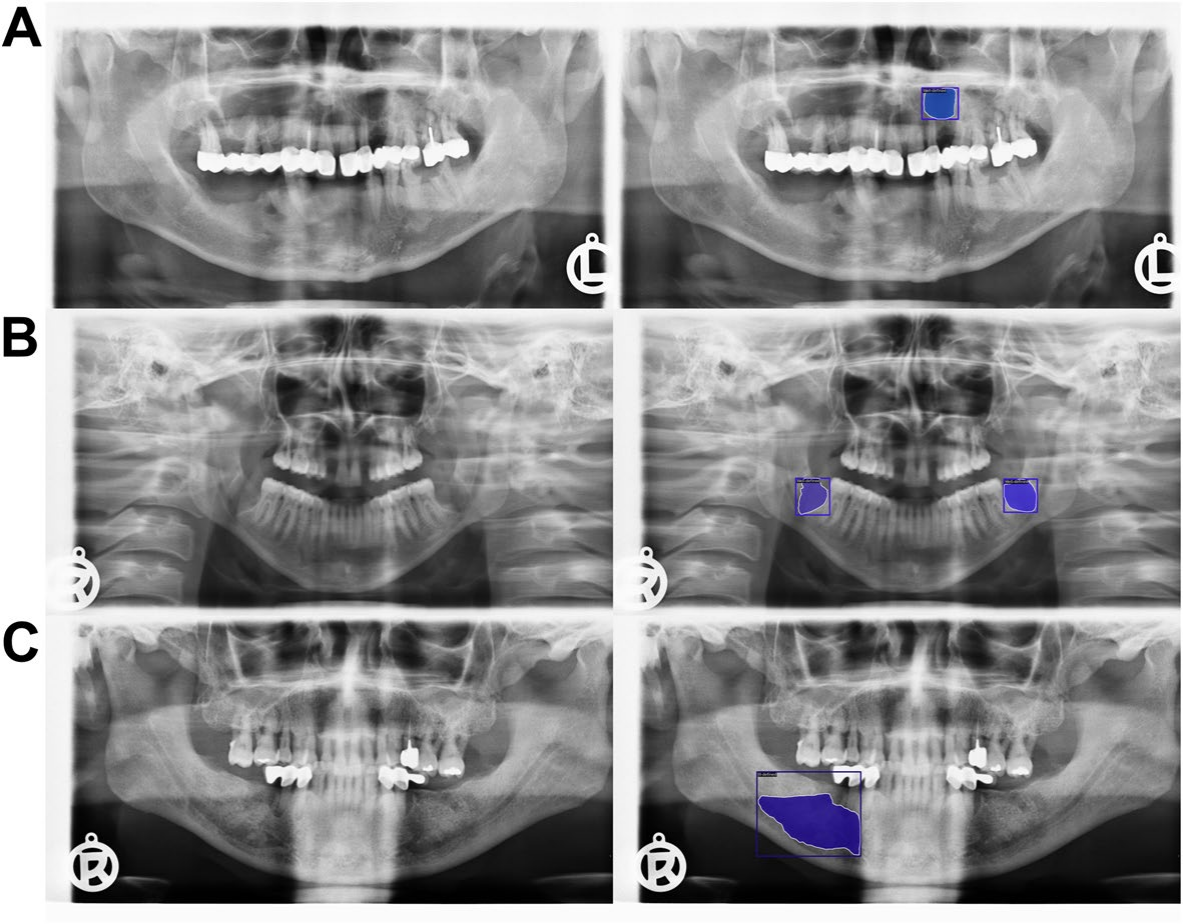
Examples of False-positive predictions. The panoramic radiographs (PRs) in the left column show cases where the most effective model—Mask R-CNN with a Swin-Tiny backbone—falsely predicted osteolytic lesions, as displayed in the right column. In image A, the model incorrectly identified a lesion in the upper jaw near the maxillary sinus. Image B shows false positive detections at sites of recent tooth extractions. In image C, an imaging artifact was mistakenly annotated as a lesion. These examples highlight common sources of false positives, including anatomical variations, extraction sites, and radiographic artifacts
